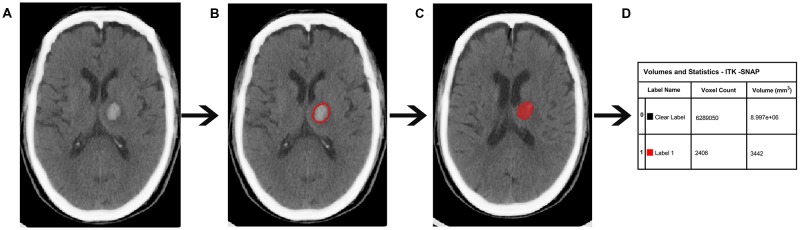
Quantitative measurement of intracerebral hemorrhage (ICH) volume. (A) Original computed tomography (CT) image. (B) The high-density area. (C) A sketch of the high-density area. (D) Calculation of the ICH volume.
